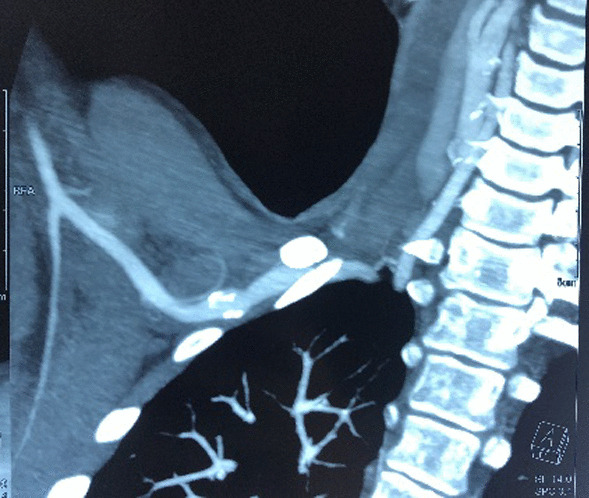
Right subclavian artery abnormalities on computed tomography angiography
